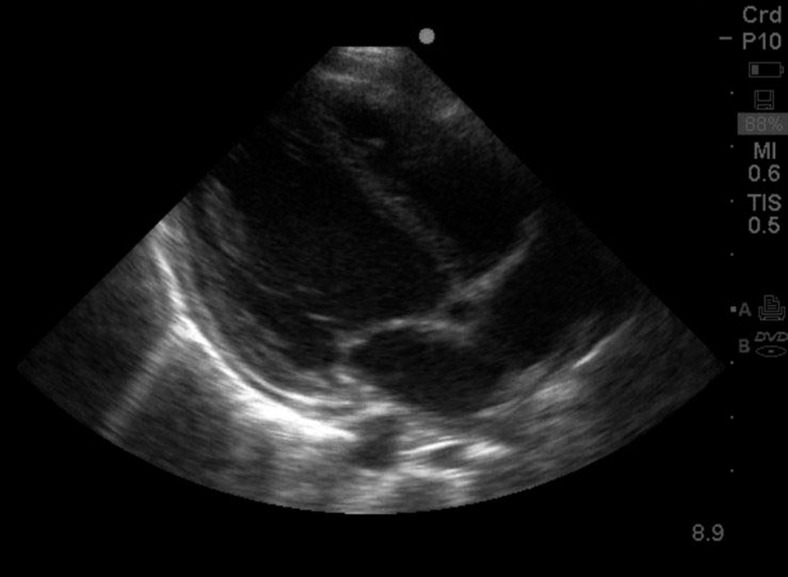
Repeat parasternal long axis view showing resolution of pericardial effusion.
